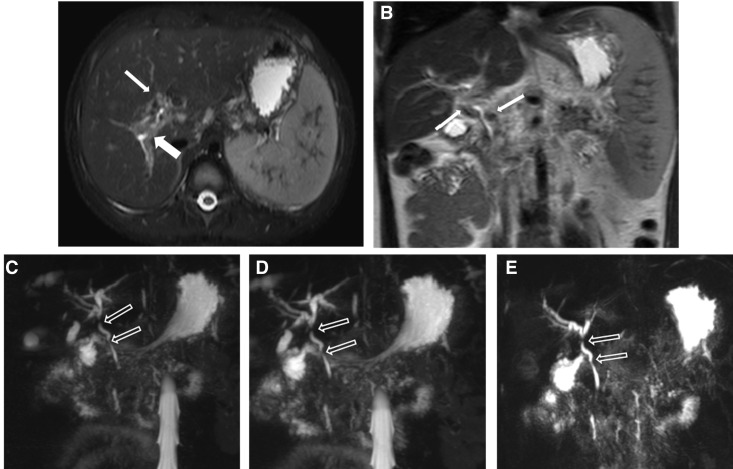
A concentric low–signal intensity area is seen surrounding the bile ducts, suggesting epicholedochal veins and fibrosis on axial fat-saturated T2-weighted imaging (A) (thick arrows), corresponding to signal-void collateral vessels (thin arrows) on axial fat-saturated T2-weighted (A) and coronal T2-weighted images (B). MRCP images (C–E) demonstrating narrowing of the common bile duct (CBD) and common hepatic duct (open arrows), with bile duct dilation. Axial fat-saturated T2-weighted imaging (A), coronal T2-weighted image (B), MRCP images (C, D, E).
